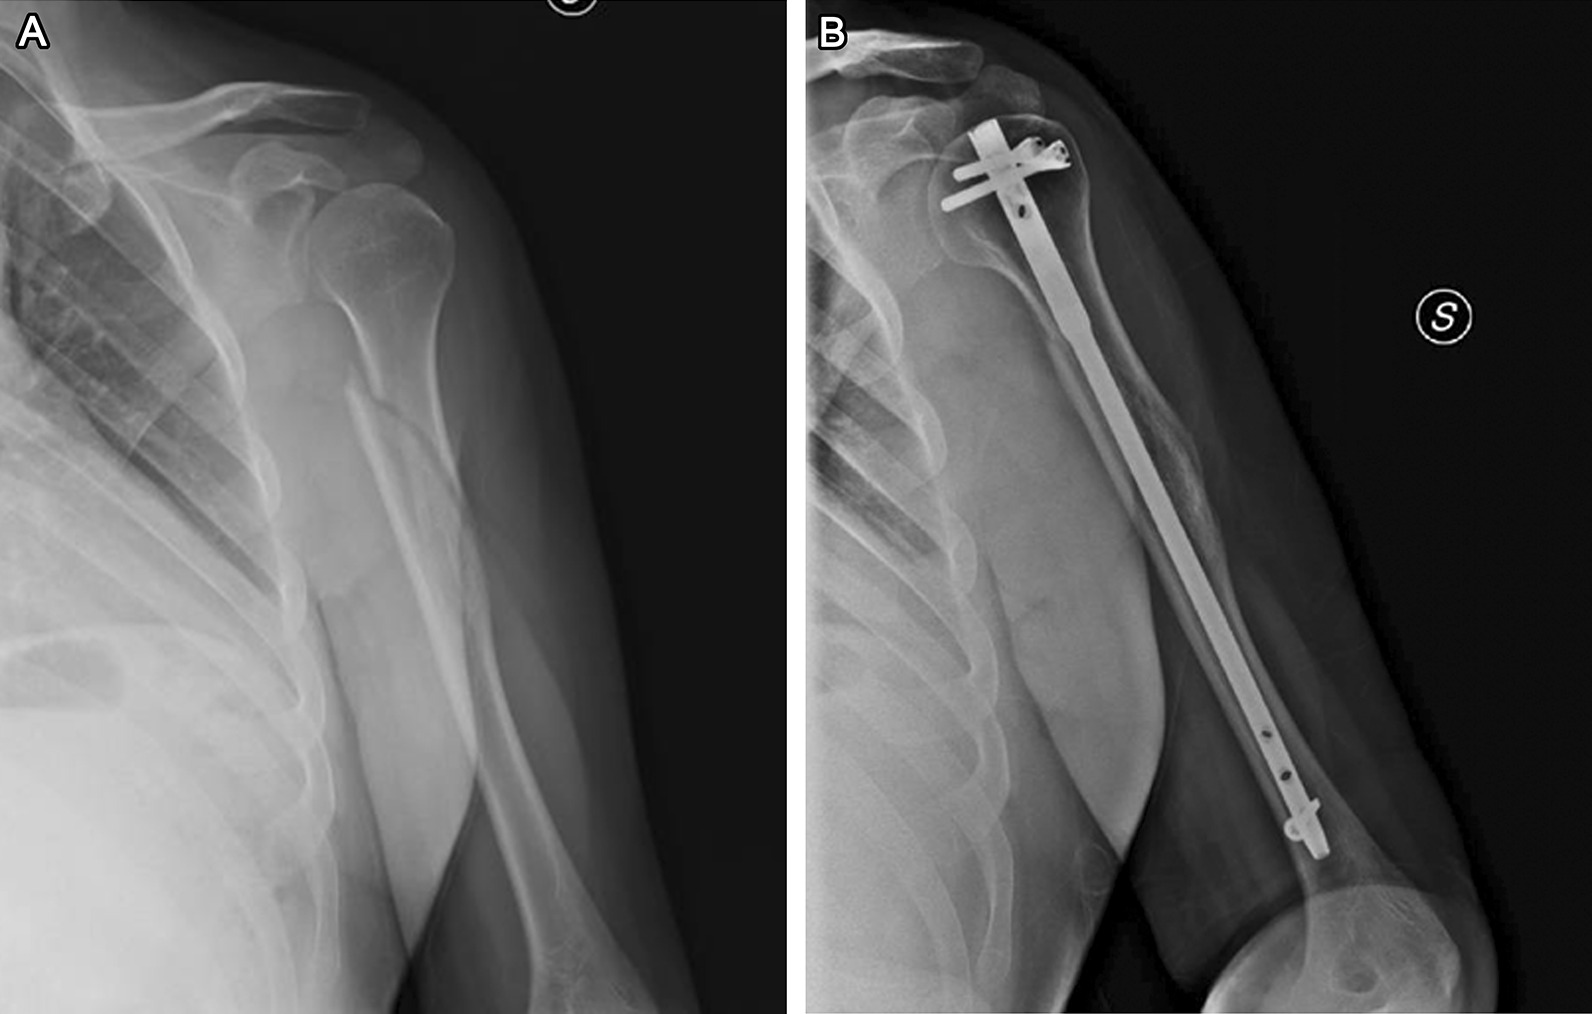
A Radiograph shows a spiral fracture of humeral diaphyseal segment (AO cl. 12A1). B Good fracture healing at 180 days post-op after osteosynthesis with a straight design intramedullary nail
